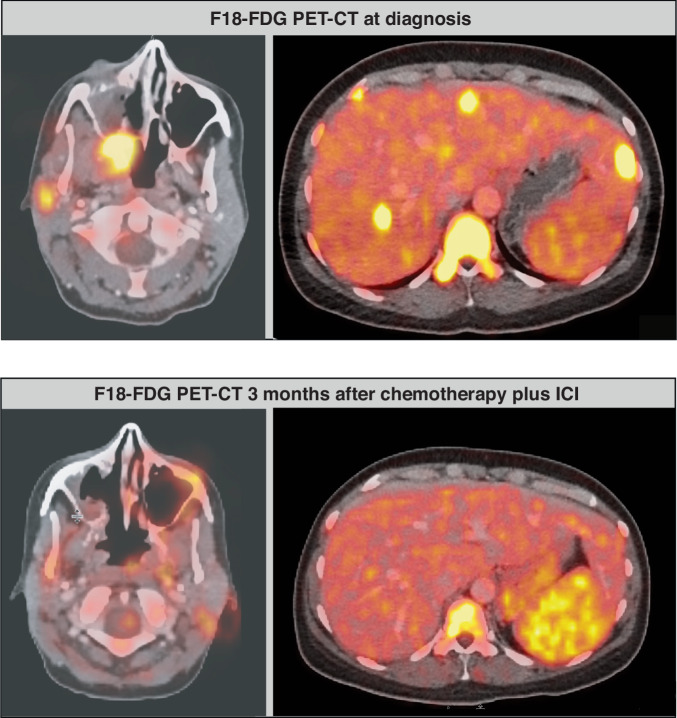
Interim whole-body F18-FDG PET-CT. Whole-body F18-FDG PET-CT staging revealed initial tumor regression and decreased metabolic uptake of the primary nasopharyngeal tumor, as well as of the liver metastasis (bottom), compared to the initial staging at diagnosis (top).
